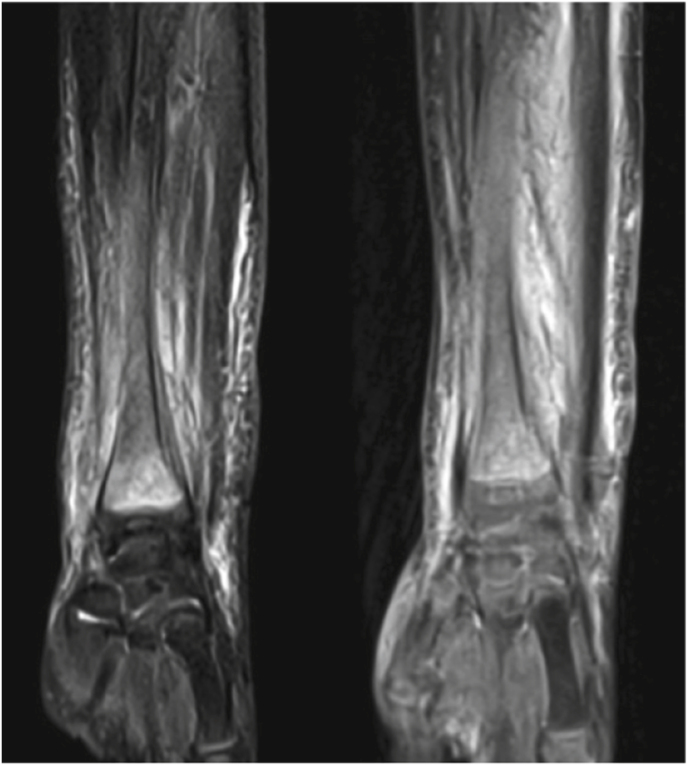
MRI of the Right forearm demonstrating cortical irregularity of the distal radius and ulna with surrounding soft tissue edema consistent with osteomyelitis.
